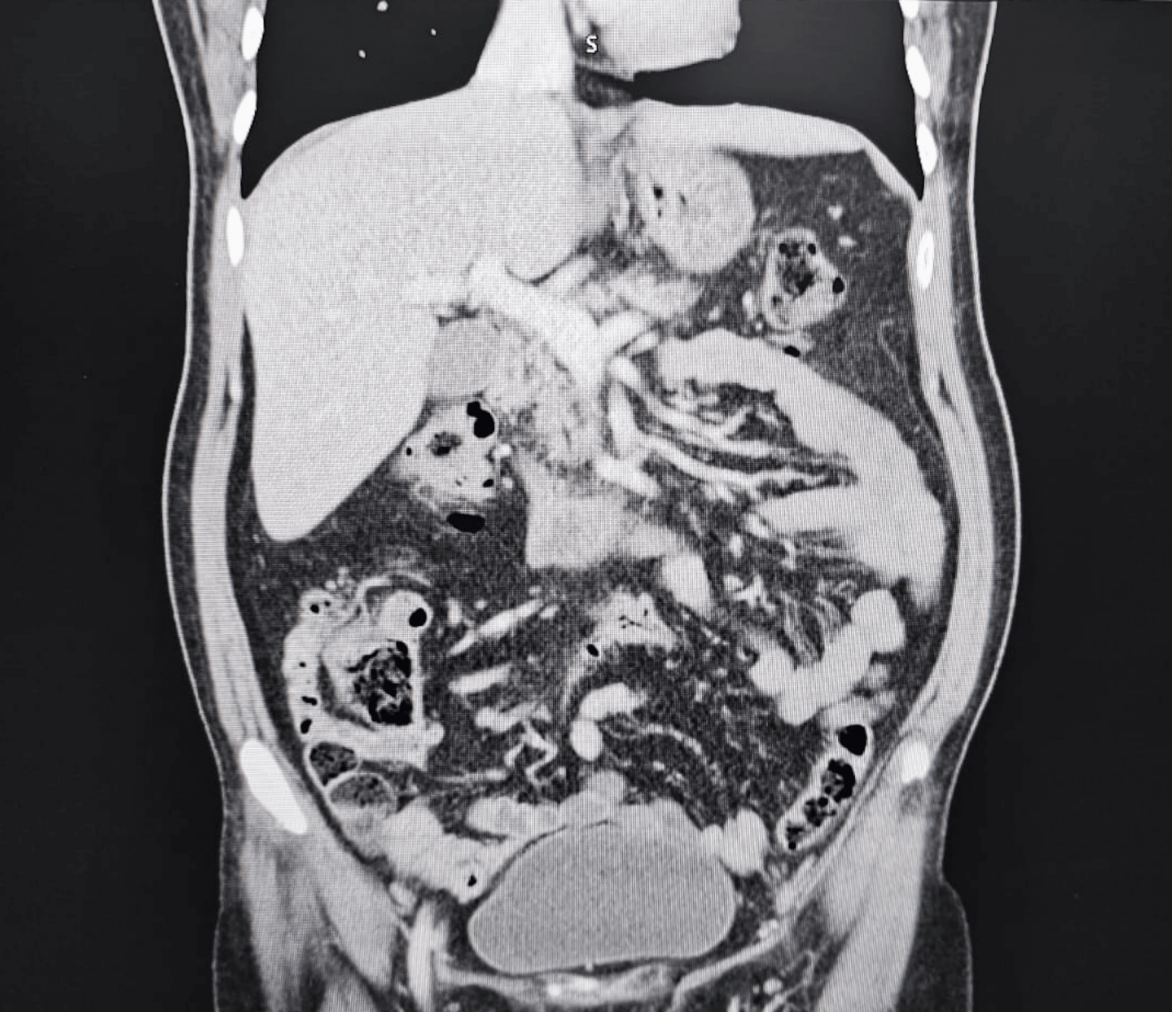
CT of the abdomen showing features of chronic pancreatitis with main pancreatic duct dilatation and head calcification. Contrast-enhanced axial CT image of the abdomen showing diffuse pancreatic parenchymal atrophy and peripancreatic fat stranding. A punctate calcification is visualized in the pancreatic head along the main pancreatic duct. The duct is markedly dilated (≈9 mm) proximally with abrupt distal narrowing at the ampullary region and subtle peripheral wall enhancement. No evidence of pancreatic collection, mass lesion, or ascites is seen. Imaging features are characteristic of established chronic pancreatitis with ductal pathology.
